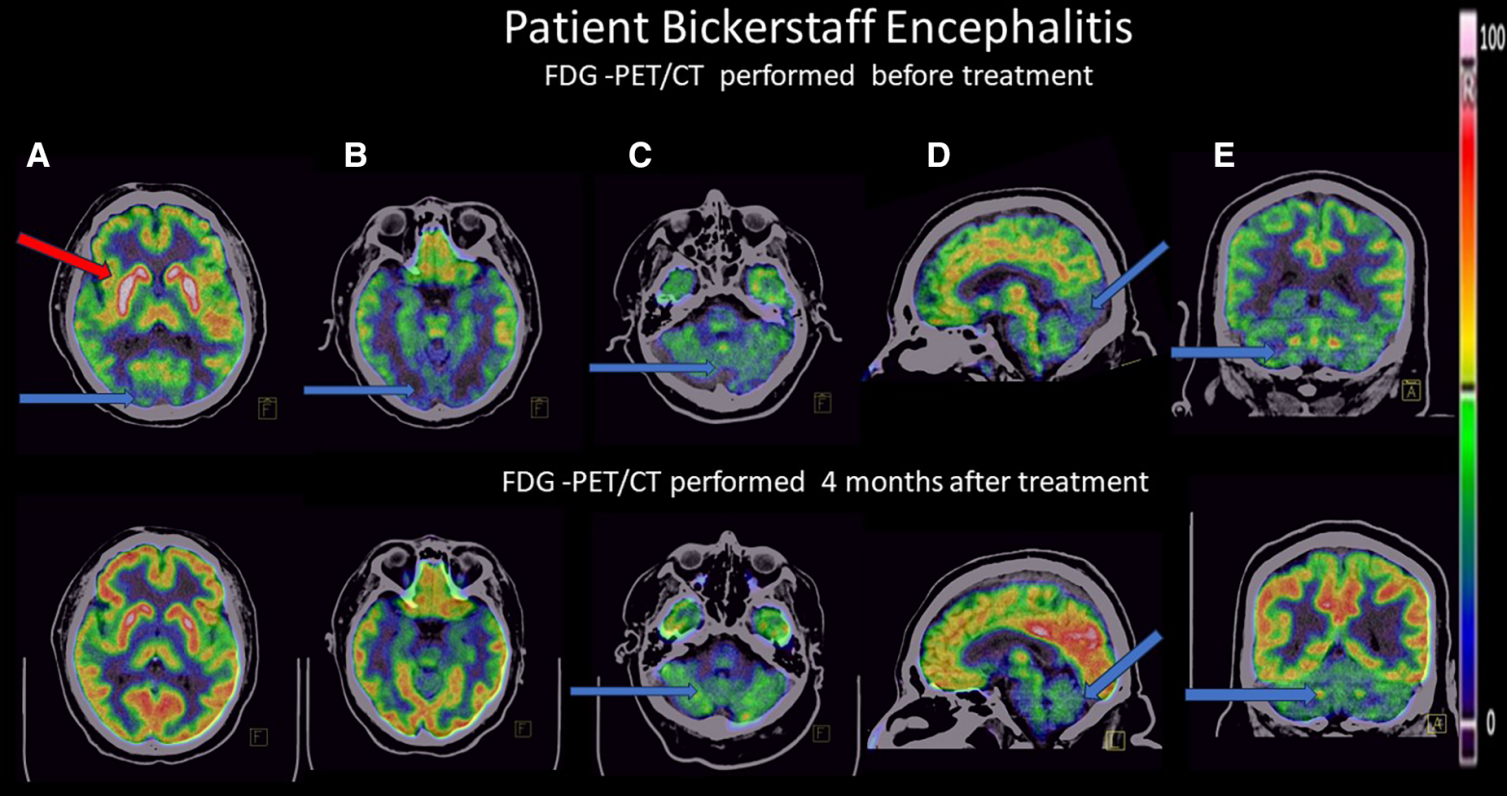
Positron emission tomography (18PET-FDG) brain images from the patient with BBE in 3 orientations (axial, coronal, sagittal views), upper images in the acute phase, lower images 4 months after treatment. Blue arrows show hypometabolism, red arrows show hypermetabolism. Thalamus was used as a reference area for normalization due to its preserved glucose metabolism during this disease. (A) Axial view: upper image: mild generalized cortical hypometabolism, severe bilateral striatum hypermetabolism, and severe bilateral occipital hypometabolism; lower image: normalization of FDG metabolism in these areas. (B) Axial view: upper image: severe bilateral occipital hypometabolism; lower image: normalization of FDG metabolism. (C) Axial view: upper image: severe bilateral cerebellar hypometabolism, lower image: discrete bilateral cerebellar hypometabolism. (D) Sagittal view: upper image: mild generalized cortical hypometabolism, severe occipital, cerebellum, brain stem, and vermis hypometabolism bilaterally; lower image: normalization of FDG pattern except for discrete cerebellar, vermis and ventral brain stem hypometabolism. (E) Coronal view, upper image: severe occipital and cerebellar hypometabolism bilaterally. Lower image: FDG normalization at the occipital cortex bilaterally, discrete bilateral cerebellar hypometabolism.
